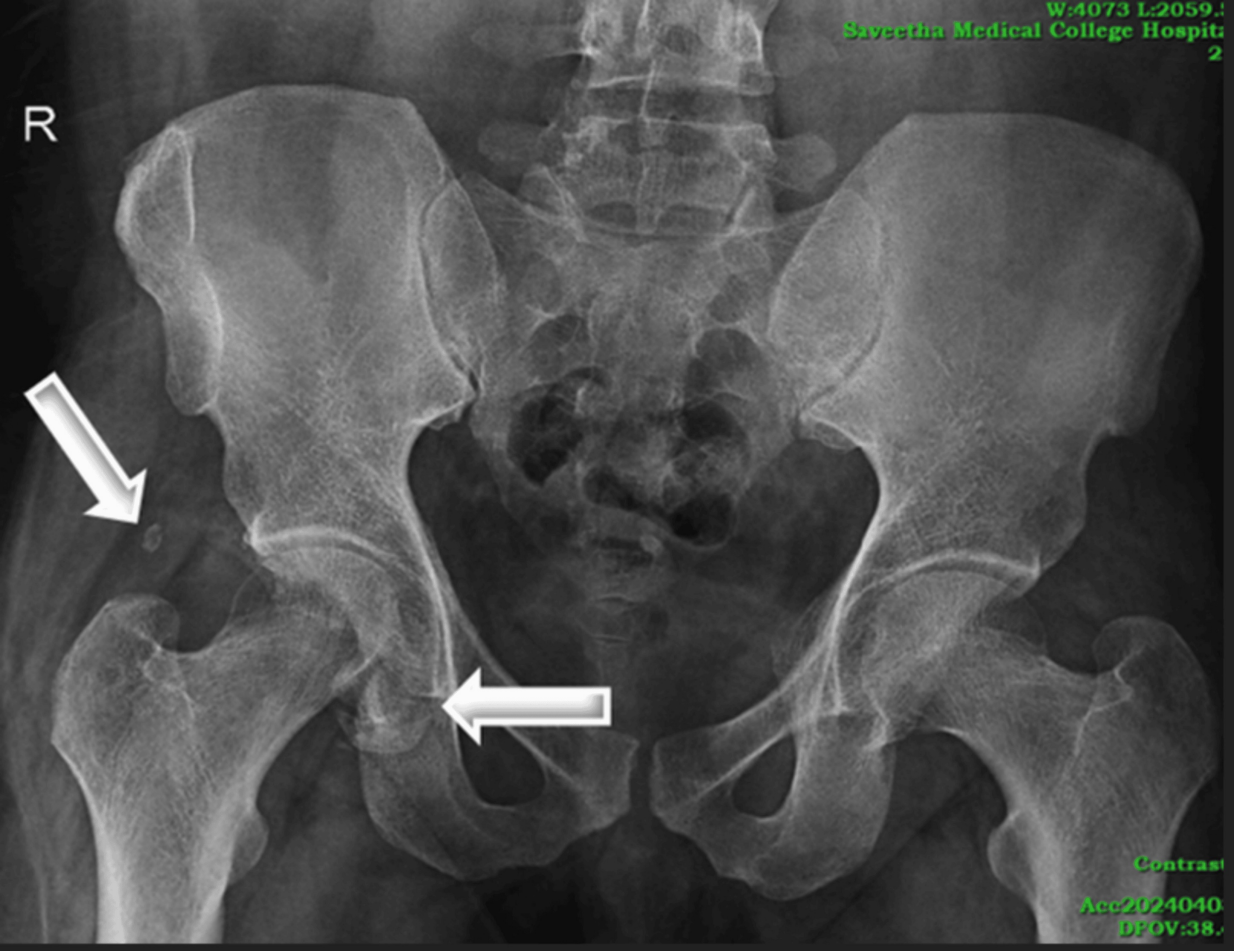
X-ray pelvis antero-posterior view showing mild disruption of Shenton's line with marked flattening of the infero-medial surface of the right femoral head with the fracture fragment displaced inferiorly and a radio-opacity noted over the greater trochanter of the right femur (likely bone fragment) represented by white arrows.
